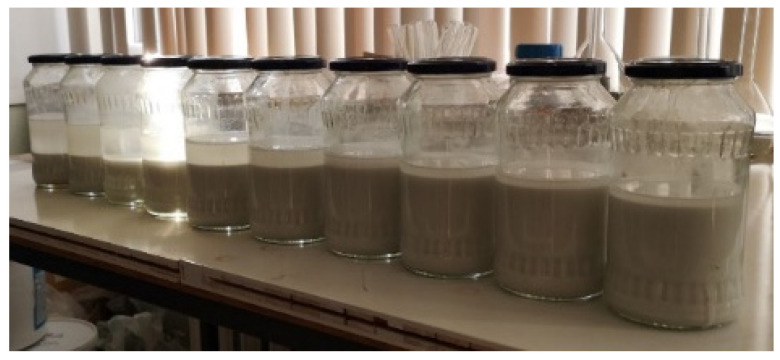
Photographs of phosphogypsum and caustic soda suspensions after ultrasonic treatment.
